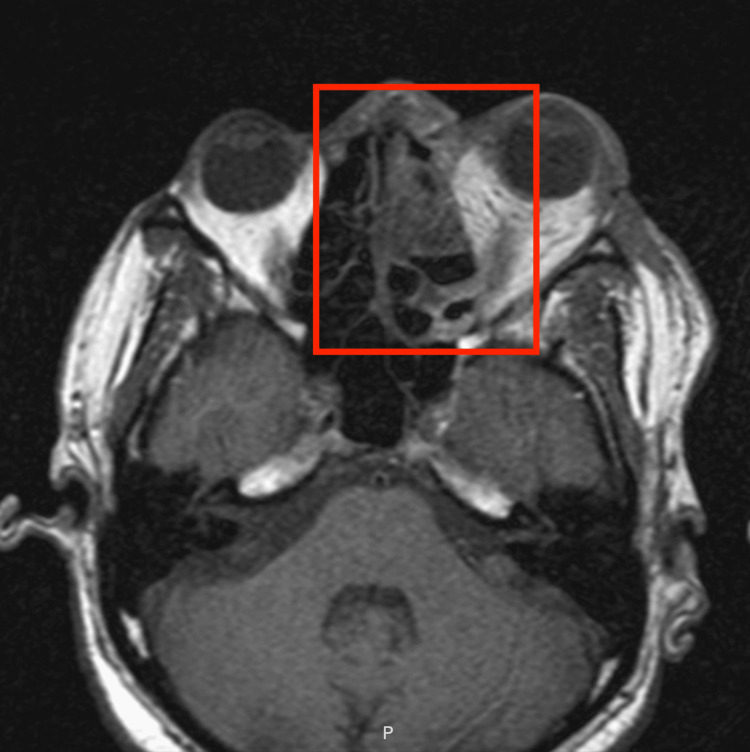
Axial contrast-enhanced MRI demonstrates non-enhancement of the left cavernous sinus, with associated left orbital cellulitis, proptosis, and the left lateral rectus muscle. A red box has been placed over the area of concern.
